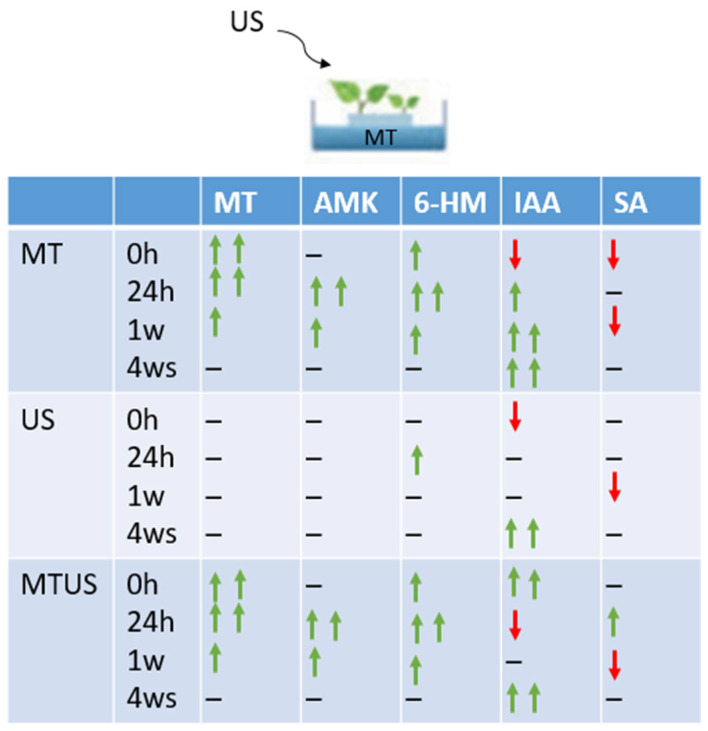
Summary figure of the measured analytical parameters. Abbreviations: Column: melatonin-containing medium (MT), ultrasound treatment (US) and combined treatment. Row: Melatonin (MT), N-acetyl-5-methoxykynuramine (AMK), 6-hydroxymelatonin (6HM), 5-methoxytryptamine (5MTA), indole-3-acetic acid (IAA), salicylic acid (SA). Green arrow—increase; red arrow—decrease.
